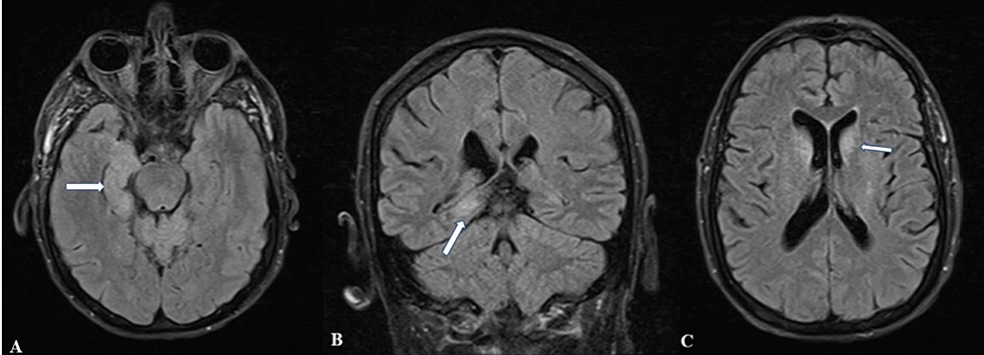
Initial MRI of the brain. MRI of the brain showing FLAIR hyperintensities in the right temporal lobe (panel A and B) as well as in the bilateral caudate nucleus (panel C). FLAIR: Fluid-attenuated inversion recovery.
